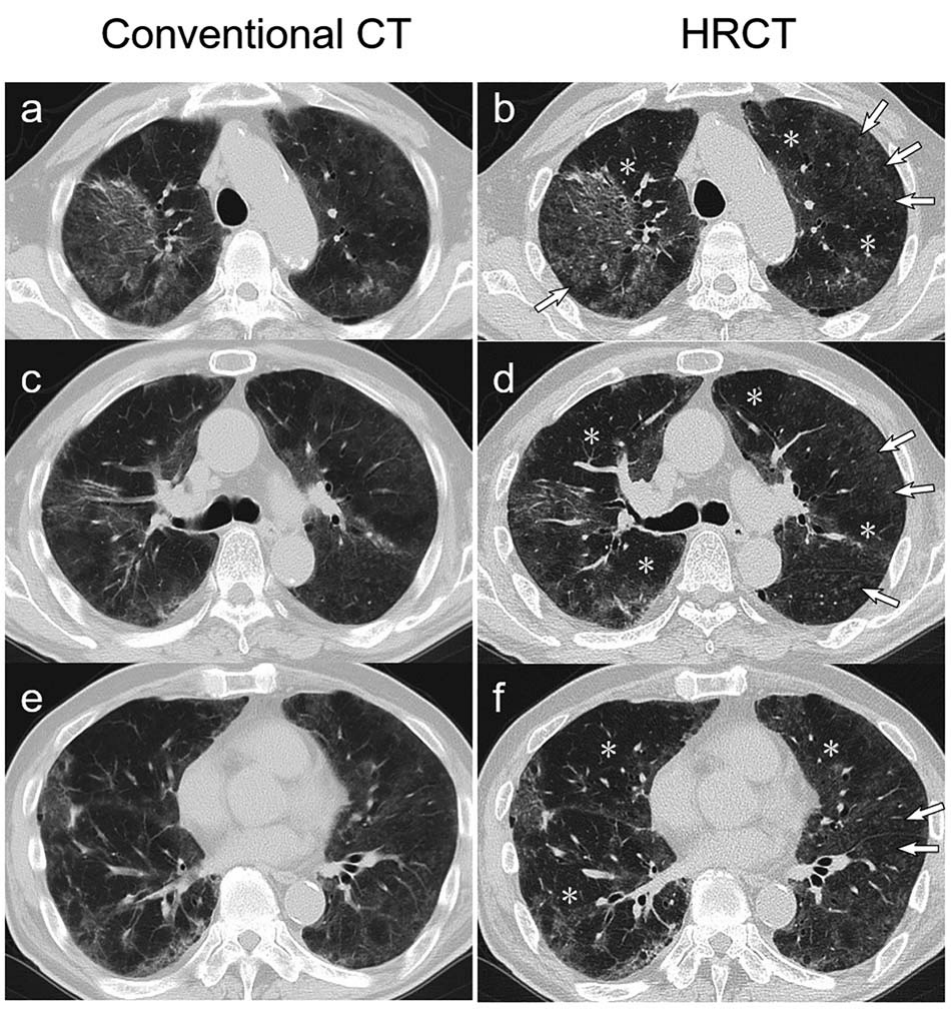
Chest CT scan images taken during hospitalization. A conventional 5-mm-thick CT scan (a, c and e) and high-resolution 2-mm-thick CT (HRCT) scan (b, d and f) taken at the levels of the aortic arch (a and b), trachea carina (c and d) and aortic valve (e and f) show diffuse ground-glass opacities (GGOs) with relatively predominant peripheral distribution on the bilateral lungs. The HRCT scan shows ill-defined centrilobular nodules (*arrows*) and mosaic attenuation (*asterisks*), which are characteristics of hypersensitivity pneumonitis.
